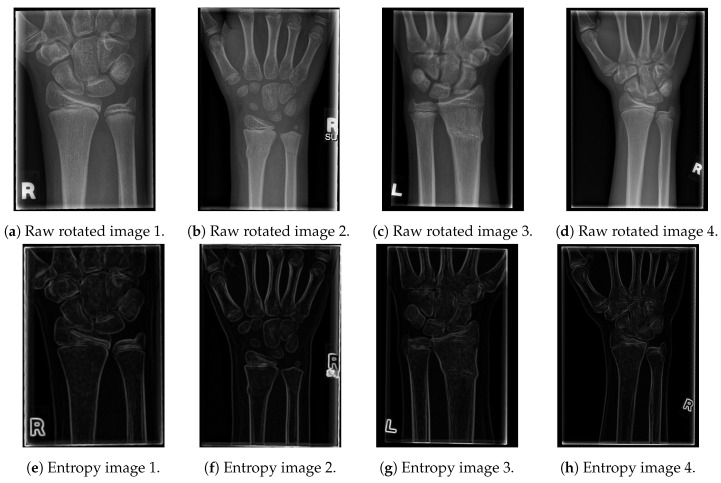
The results of the proposed entropy algorithm on X-ray images.
